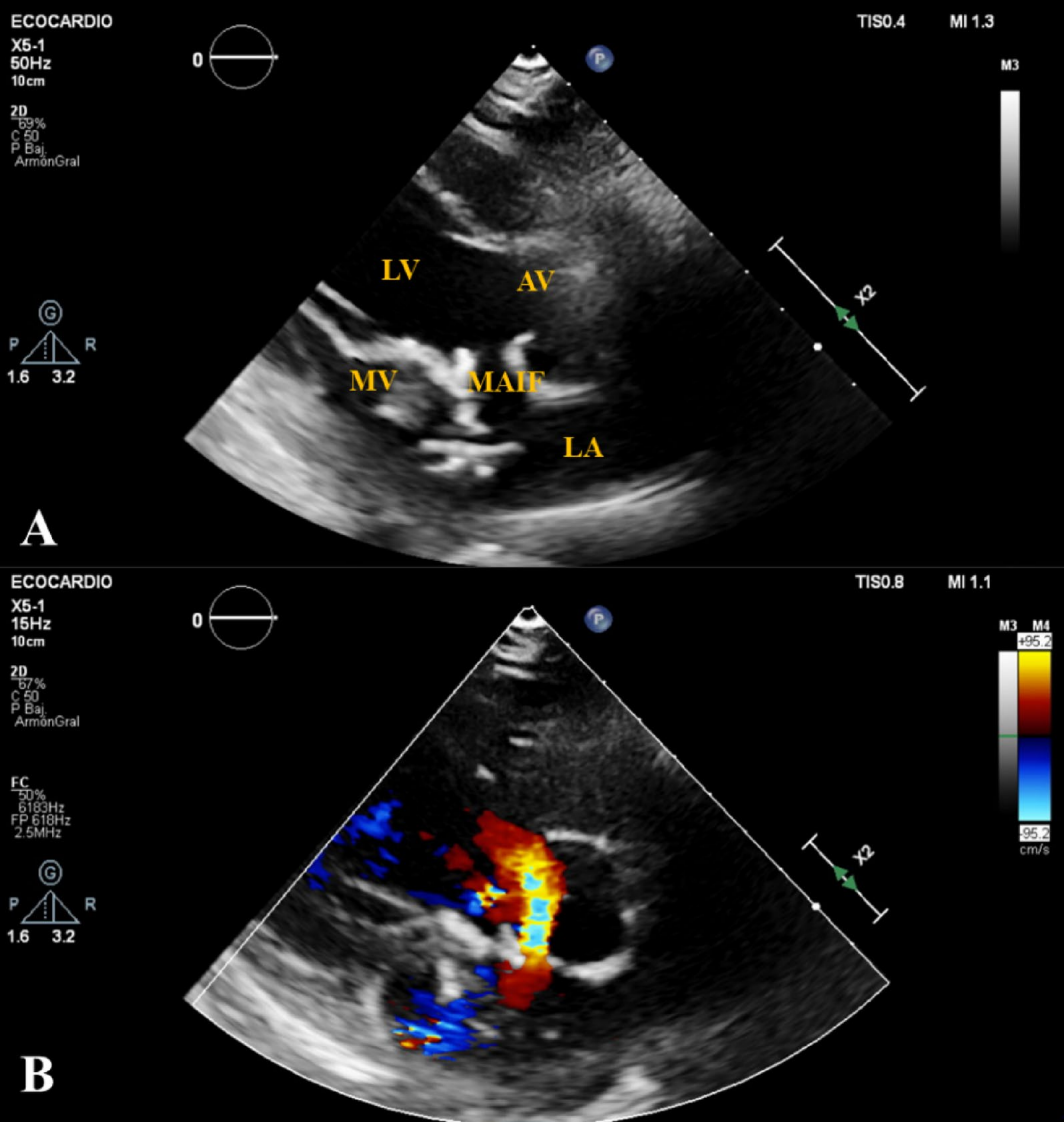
A An aberrant communication between the mitral valve and the aortic valve, corresponding to the rupture of the MAIF, is observed. B An abnormal flow through the MAIF is detected using color Doppler echocardiography. *LA* Left atrium, *MV* Mitral Valve, *LV* Left Ventricle, *AV* Aortic Valve
